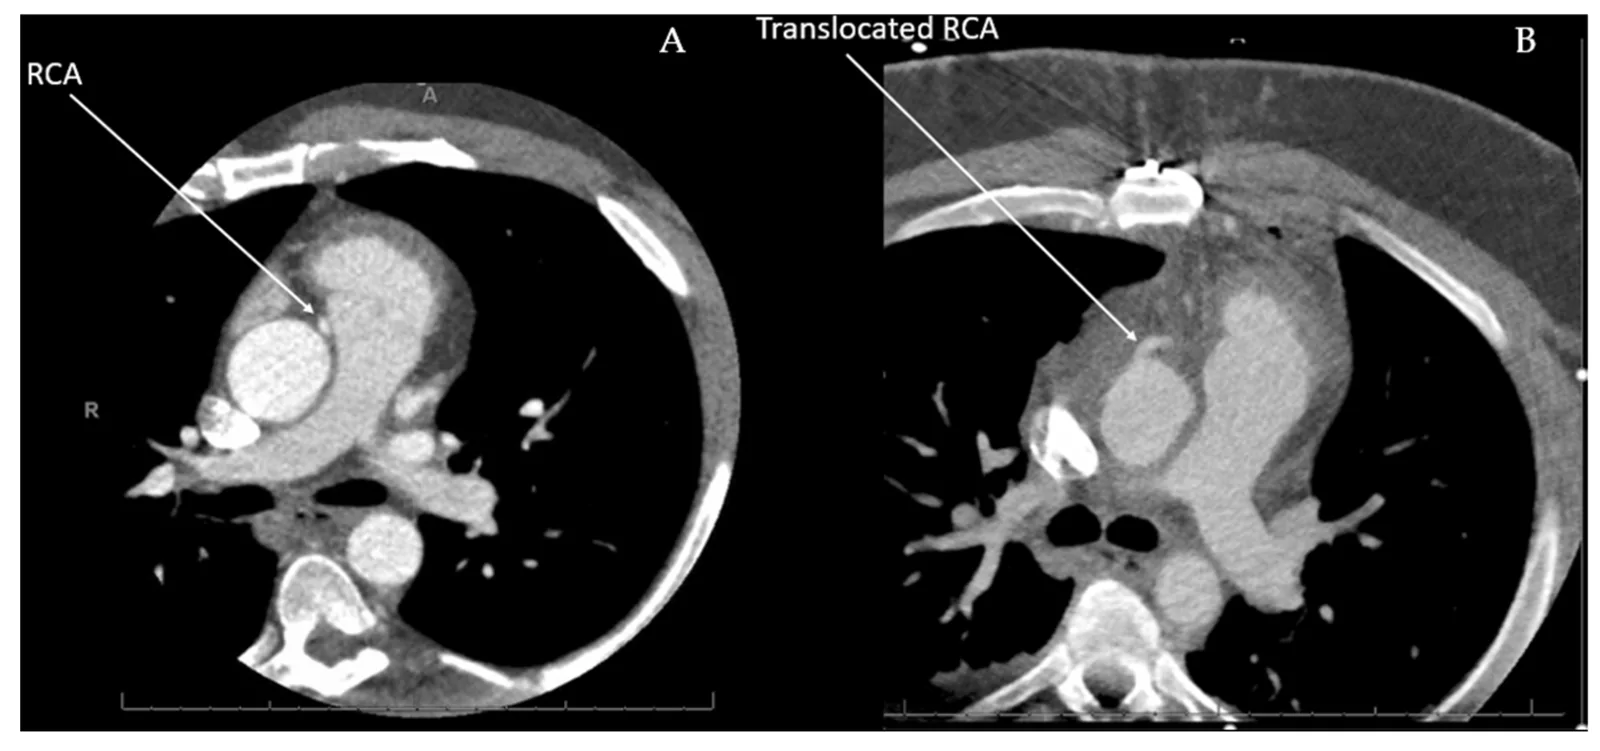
(A,B): If the anomalous coronary artery does not have an intramural segment, then translocation of the entire ostium can provide an alternate solution. (A) preoperative and postoperative (B) computed tomography scan images showing the inter-arterial course of the right coronary artery (RCA) with no intramural segment and in (B) after being translocated to the ascending aorta; RCA: right coronary artery.
